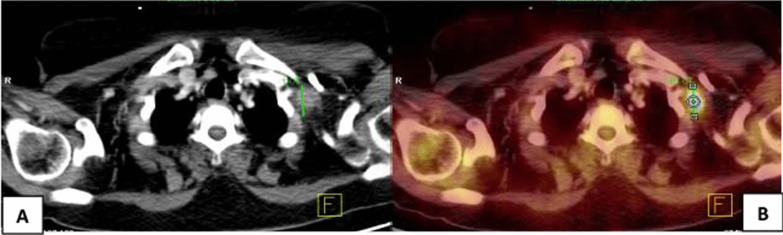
A 50-year-old female patient with NHL, treated with anti-cd20 antibody rituximab 5 months prior to vaccination. She was evaluated for left supraclavicular pain. Clinical data revealed that she had received the 2nd dose of Pfizer-BioNTech COVID-19 vaccine in her left arm 8 days prior to the PET/CT study. A Axial CECT image revealed enlarged left supraclavicular LN 2.6 cm. B Axial PET/CT image revealed enlarged FDG-avid left supraclavicular LN of 2 SUVmax
